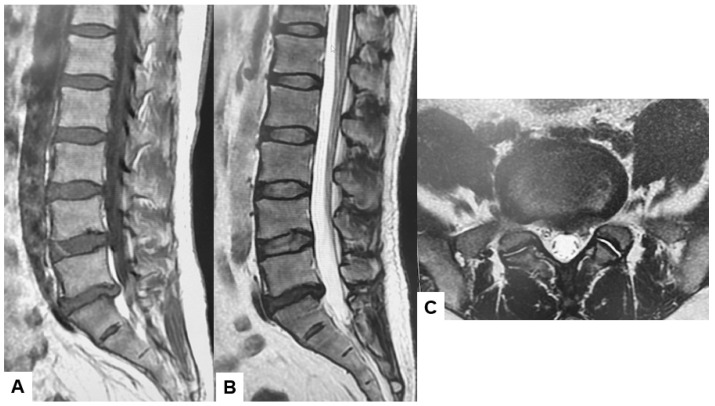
Forty-two-year-old M, left L5/S lumbar disk herniation, (A) T1-weighted midsagittal image, (B) T2-weighted midsagittal image, (C) T2-weighted axial image at L5/S1. Lumbar disk herniation (median and paramedian) is indicated at left L5/S1.
